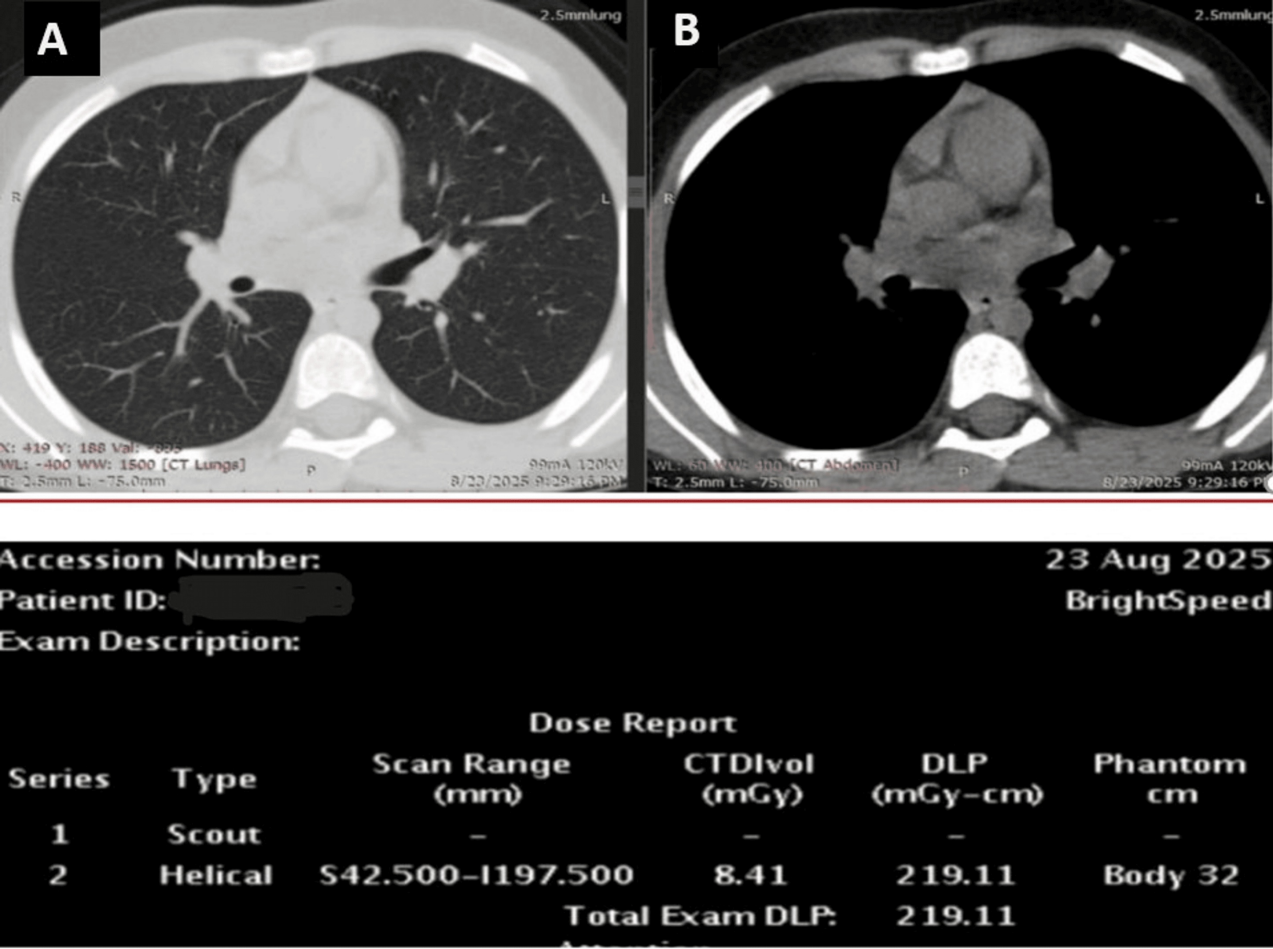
Another axial chest CT scan shows images in lung (A) and soft (B) tissue windows. A reduction in DLP is observed following the parameter adjustment
